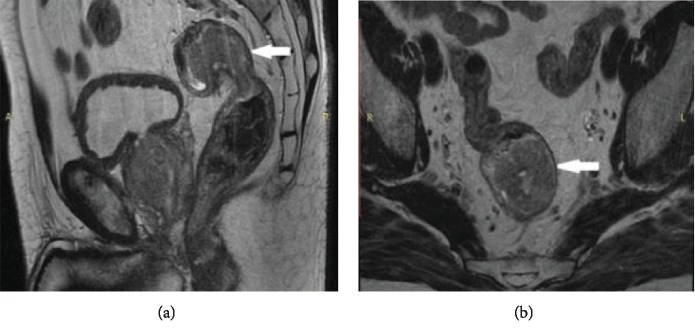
Sagittal (a) and axial (b) reconstruction of a T2-weighted MRI showing the rectal tumor (arrow).
